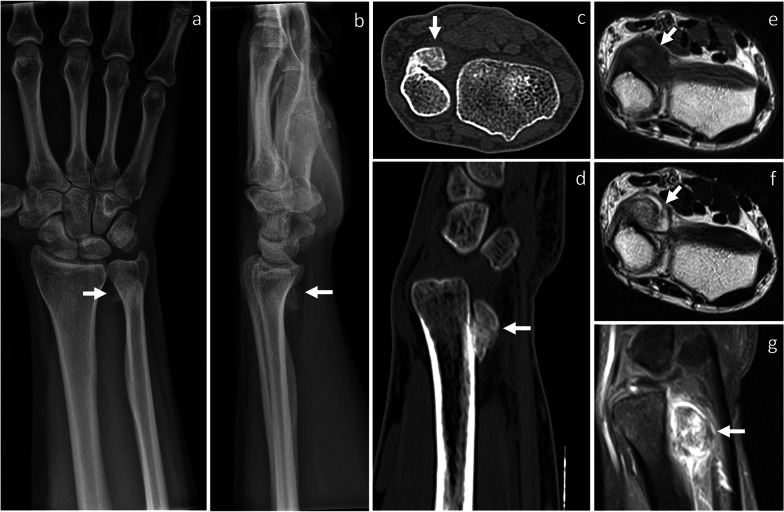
BPOP arising from the distal ulnar metaphysis. On X-rays, frontal (a) and lateral (b) views show a well-defined mass of heterotopic mineralization, which is contiguous to the palmar aspect of the distal ulnar metaphysis. On axial (c) and sagittal (d) CT images, the mass is cortex-based with no cortico-medullary continuity, cortical breakthrough, or marrow extension. On axial T1-weighted (e) and T2-weighted (f) MRI sequences, the mass shows low-to-intermediate and high signal, respectively. After contrast administration, marked contrast enhancement is seen on sagittal fat-saturated T1-weighted sequence (g). After biopsy, surgical resection was performed and BPOP was pathologically proven. Arrow points at BPOP in all images
